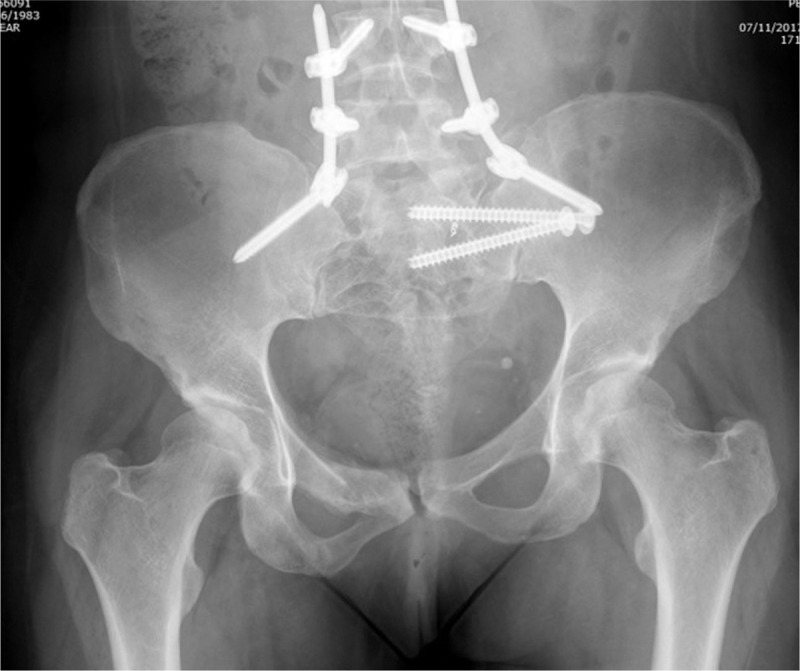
The complete fixation as seen in a postoperative radiograph obtained at 3 mo postoperatively. The patient is pain free and weight bearing.
